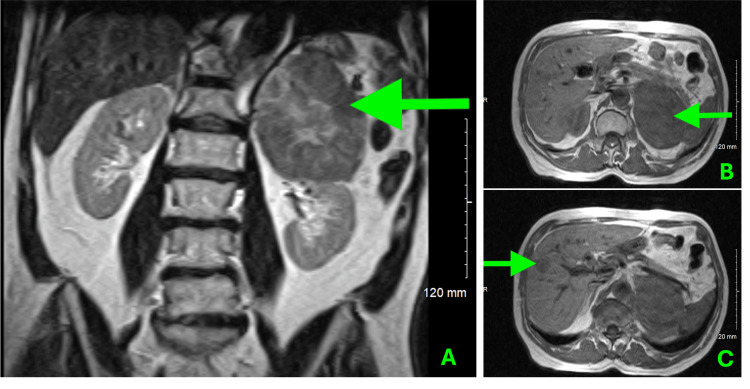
MRI Imaging A. T1-weighted coronal MRI slice shows a large mass in the left adrenal gland indicated by the green arrow. B. Axial MRI slice demonstrates the tumor's full width, green arrow indicating the adrenal mass. C. The green arrow indicates the 2.8 cm hepatic lesion observed in the right lateral segment of the liver.
